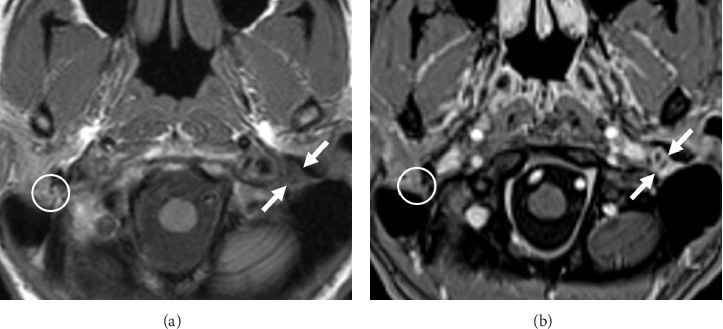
(a, b) Axial MRI T1-weighted images acquired before (a) and after (b) administration of gadolinium contrast demonstrate abnormal T1 hypointense, enhancing soft tissue (Arrows A and B) effacing the fat of the left stylomastoid foramen. The normal appearance of the right stylomastoid is marked (Circles A and B) for comparison. In conjunction with the CT scan findings, the enhancing soft tissue was considered most likely to represent inflammation and granulation tissue forming in reaction to pathologic motion at the left styloid process pseudoarthrosis.
